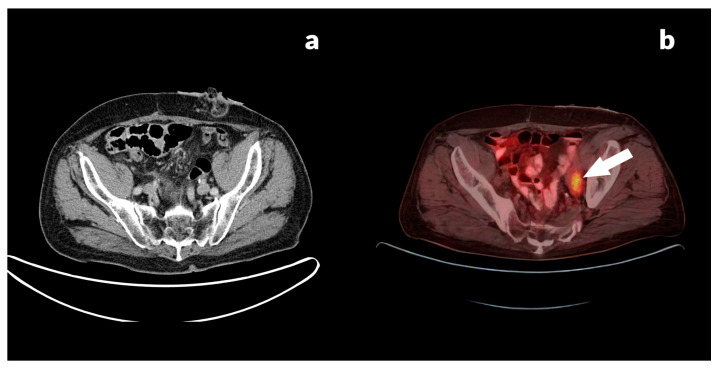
(a) Diagnostic CT in axial plane shows no lesion in the left pelvic region, and (b) PET/CT axial image detects an FDG-avid ovoid lesion in the left obturator area, SUVmax 7.20 (white arrow), corresponding with lymph node involvement.
